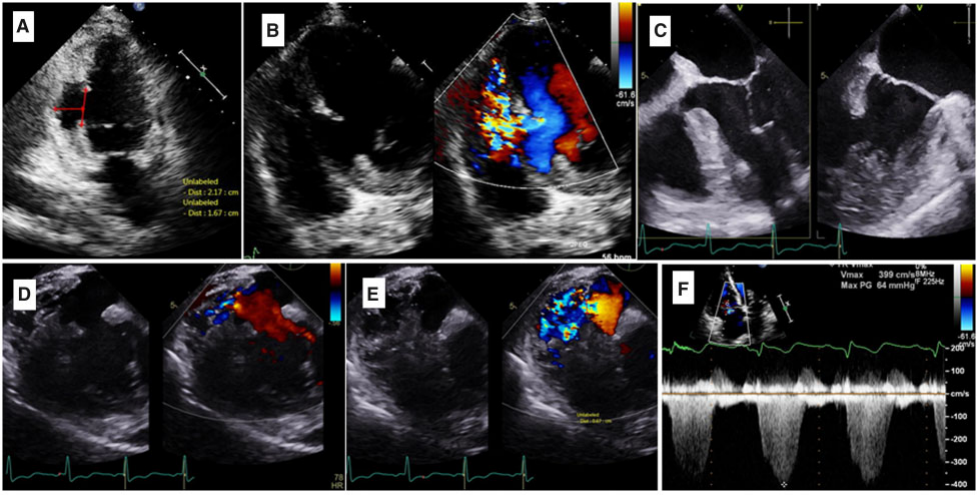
(*A*) Transthoracic echocardiogram apical two-chamber view showing inferior wall true aneurysm. (*B*) Transthoracic echocardiogram modified apical view showing inferior septum ruptured and dissected with left-to-right shunt. (*C*) Transoesophageal echocardiogram X-plane showing the inferior wall aneurysm. (*D*) Transoesophageal echocardiogram transgastric short-axis view in diastole showing the inferior wall aneurysm with a wide neck. (*E*) Transoesophageal echocardiogram transgastric short-axis view in systole showing the inferior wall aneurysm communication with right ventricle. (*F*) Mild tricuspid regurgitation with an estimated systolic pulmonary artery pressure of 75 mmHg.
